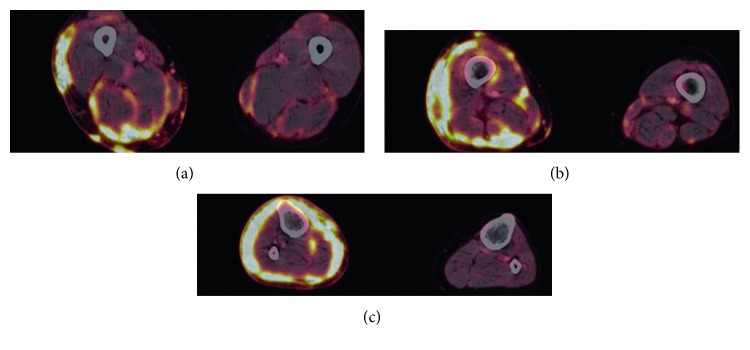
PET-CT showed pronounced inflammatory cutaneous changes of the entire circumference of the right leg and of fasciae surrounding several muscles. Minor inflammatory changes of the subcutis and muscle fasciae of the left leg are also present. (a) Upper part of thigh. (b) Lower thigh. (c) Upper part of lower leg.
